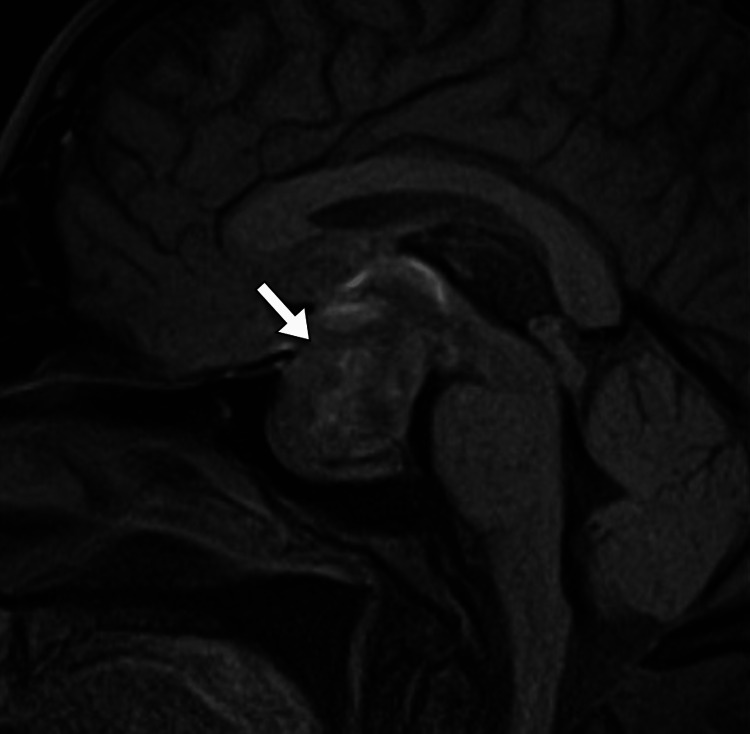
Sagittal MRI T1-weighted image shows a large intrasellar mass (arrow) enlarging the sella turcica and expanding into the suprasellar cistern MRI: magnetic resonance imaging
